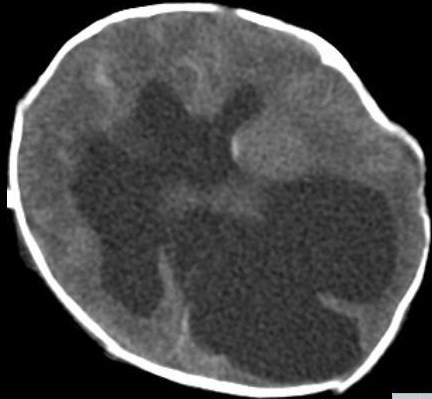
CT scan of head, axial view; showing single large monoventricle with no sylvian fissure with extensive hydrocephalus and possibility of dorsal cyst.
